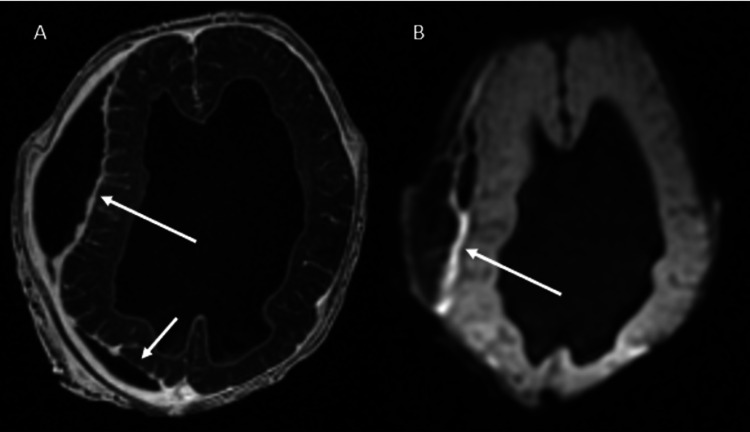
Subdural Empyema at Seven Weeks Axial post-contrast MRI of the brain (A) shows right-sided subdural fluid with peripheral enhancement (arrow). Axial diffusion-weighted MRI (B) shows restricted diffusion (arrow) within the right-sided subdural fluid consistent with subdural empyema.
